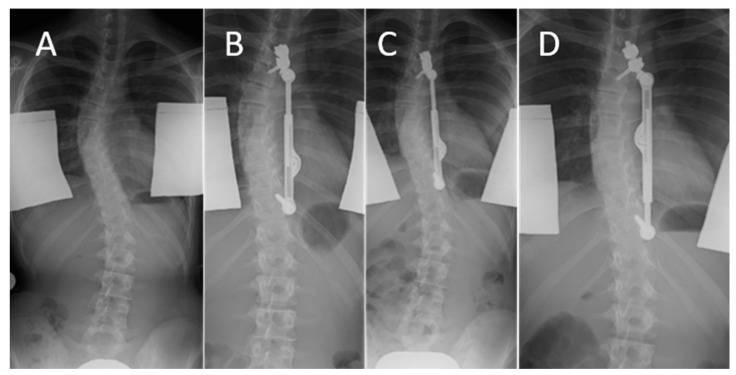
Follow-up of an 115 mm ApiFix implant. Preoperative radiograph (A), initial ApiFix device after implantation (B) and with a fully utilized distraction length 22 months after index surgery (C), and replacement with a 125 mm ApiFix up-side down system (D).
